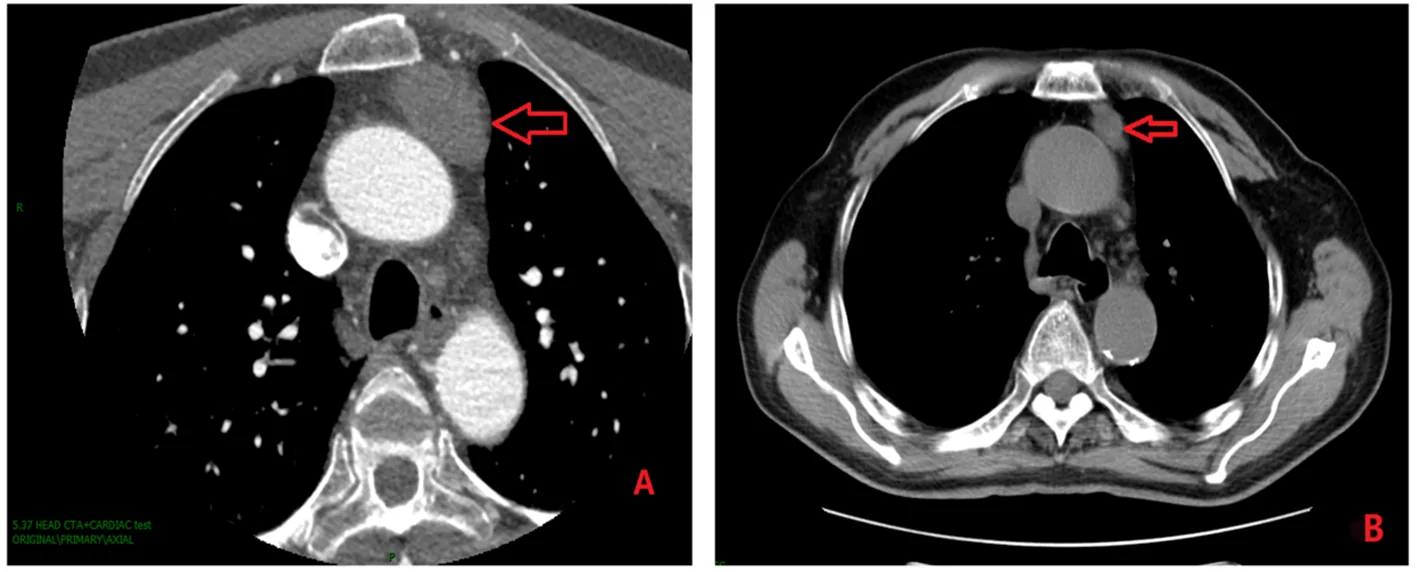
Chest computed tomography (CT) images of the patient before and after treatment with sintilimab. (A) CT image on March 5, 2022 before treatment with sintilimab. (B) CT image on May 28, 2022 after treatment with sintilimab. Lesions in the upper mediastinal soft tissue were indicated by arrows.
